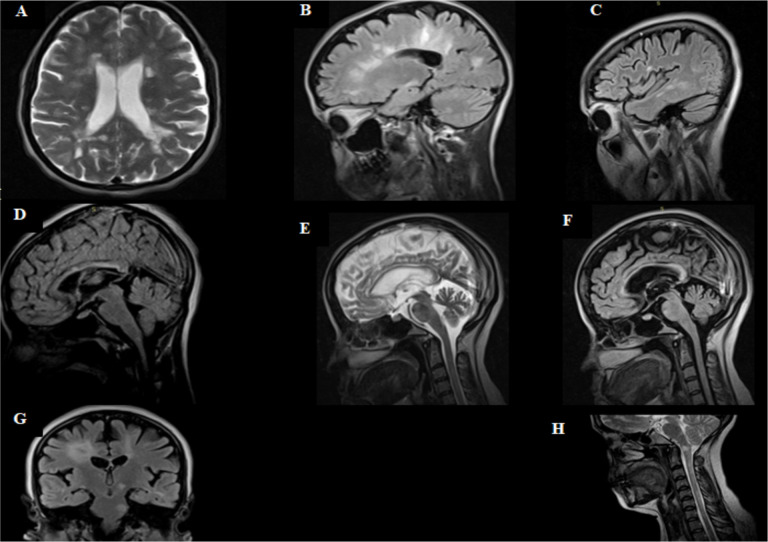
Axial T2 brain MRI showing bilateral periventricular white matter oval/round hyperintense lesions (A). Sagittal FLAIR MRI showing multiple periventricular demyelinating plaques extending radially away from the body of the lateral ventricle, Dawson's fingers (B). Sagittal FLAIR MRI showing multiple juxtacortical lesions abutting the cortex (C). Sagittal FLAIR MRI showing linear hyperintense lesion in the corpus callosum at the callososeptal interface (D). Sagittal T2 weighted image showing corpus callosal atrophy (E). Sagittal FLAIR MRI showing global brain atrophy (F). Coronal FLAIR MRI showing left cerebral peduncle and left pontine nodular hyperintensity (G). Sagittal T2 weighted image showing Rounded hyperintensity at the cervicomedullary junction and long segment T2 hyperintensity in the upper cervical cord.
